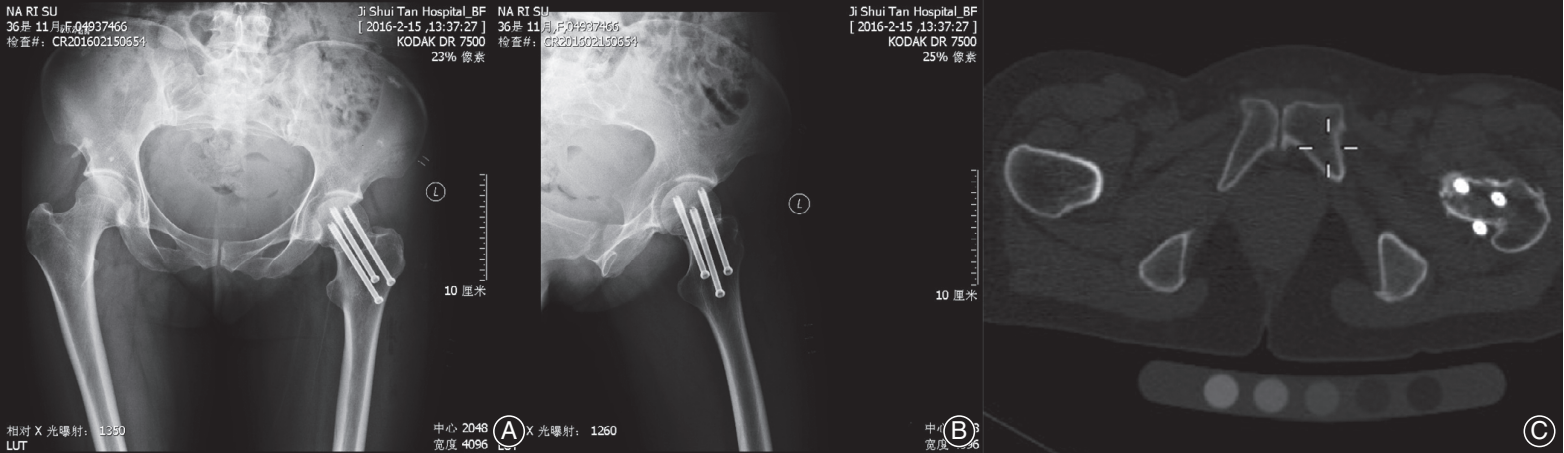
From anteroposterior (A) and lateral (B) X‐ray images, the screws appear to be in the neck of the femur, but, in fact, a screw penetrates the cortex in the posterosuperior of femoral neck (C) in the cross‐section from CT.
